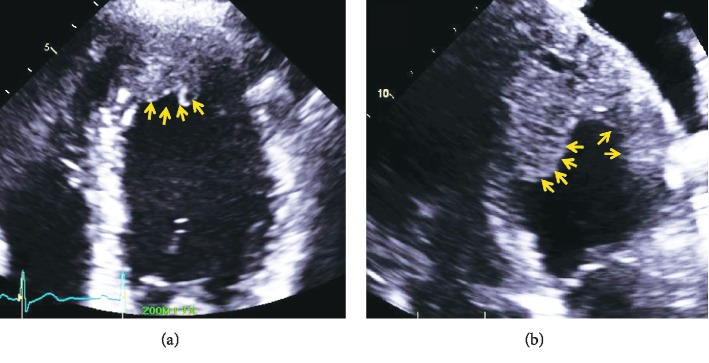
(a) Transthoracic echocardiogram shows a 4.6 × 2.8 cm mass (arrows) infiltrating the apical, antero-septal-lateral wall segments. (b) Transthoracic echocardiogram shows interval progression of the mass (arrows).
